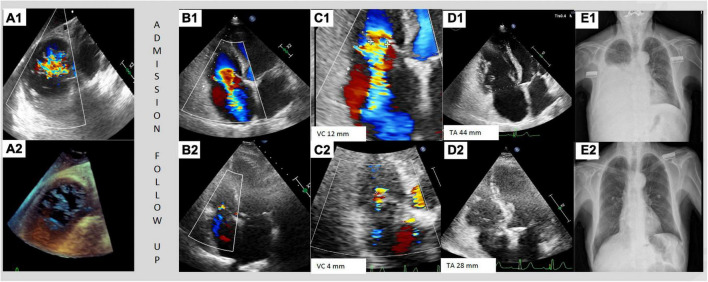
Case of dynamic changes of TR during medical therapy. Patient with previous aortic surgical replacement admitted for right heart failure and torrential tricuspid regurgitation. (A1, A2) Show the transesophageal transgastric view with torrential tricuspid regurgitation and no-coaptation between anterior and septal leaflet. (B1–E1) Images during the admission and (B2–E2) images during the follow-up with intensive diuretic treatment. The transthoracic echocardiography showed a torrential TR with a jet which filled the entire atrium (B1), vena contracta of 12 mm (C1) and a tricuspid annulus of 44 mm (D1). The thoracic radiography at admission with serious right pleural effusion (E1). Three months follow-up transthoracic echocardiography showed a mild TR (B2) with a vena contracta of 4 mm (C2) and a regression of tricuspid annulus size of 28 mm (D2). The thoracic radiography at follow-up showed no pleural effusion.
